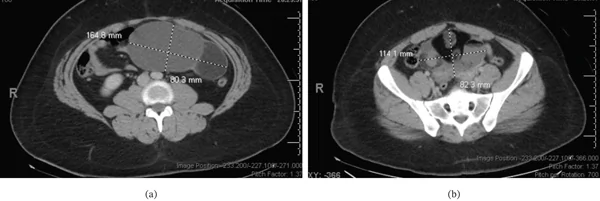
(a) Axial computed tomography of the left ovary reveals a well‐circumscribed, heterogeneous dermoid. The mass contains areas of variable attenuation, including fat densities and possible calcifications, consistent with a mature dermoid cyst. The mass exerts mild mass effect on the adjacent structures without evidence of infiltration or peritoneal free fluid. (b) Axial computed tomography of the right ovary reveals a complex, heterogeneous dermoid within the right adnexa. The mass exhibits mixed attenuation, including fat densities and possible calcifications, consistent with the characteristics of a mature cystic dermoid. The mass exerts mild mass effect on the adjacent pelvic structures with no evidence of infiltration.
